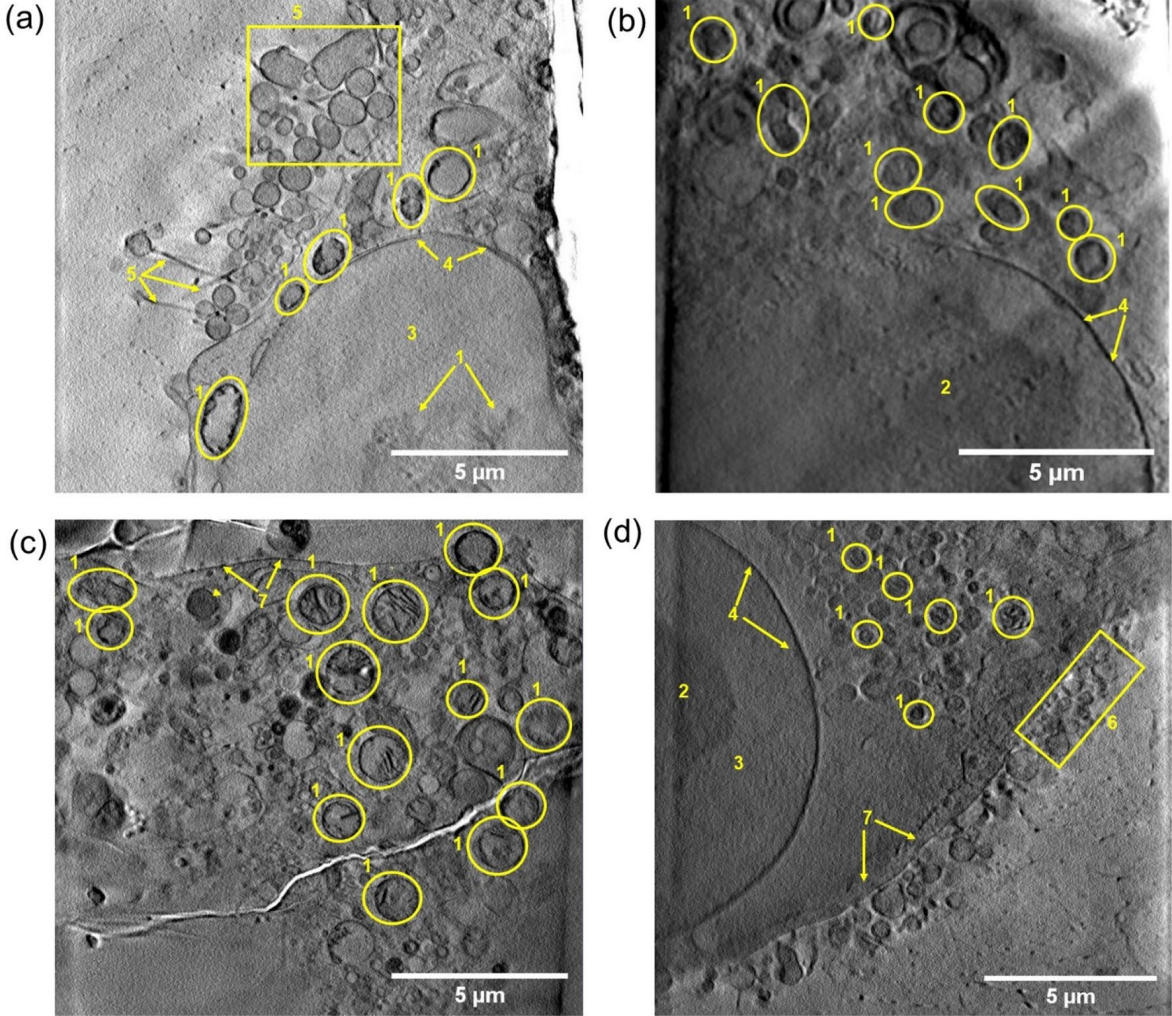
2D projections of reconstructed X-ray tomograms (16 × 16 μm^2^) of cryogenically fixed PC3 human prostate carcinoma cells grown on Quantifoil TEM grids showing changes in cellular and organelle morphology upon treatment with 1, followed by irradiation with blue light. a Untreated cells in dark conditions (not irradiated with blue light; 465 nm). b Untreated cells exposed to blue light (10 min, 465 nm, 4.8 mW/cm^2^). c Cells treated with 1 (1 μM) under dark conditions (not irradiated with blue light; 465 nm). d Cells treated with 1 (1 μM) for 2 h, followed by exposure to blue light (10 min, 465 nm, 4.8 mw/cm^2^). Cellular features shown: 1 = mitochondria, 2 = nucleolus, 3 = nucleus, 4 = nuclear membrane, 5 = features of lamellipodium, 6 = spherical vesicles, 7 = plasma membrane. Images were generated using IMOD software [33, 34]
